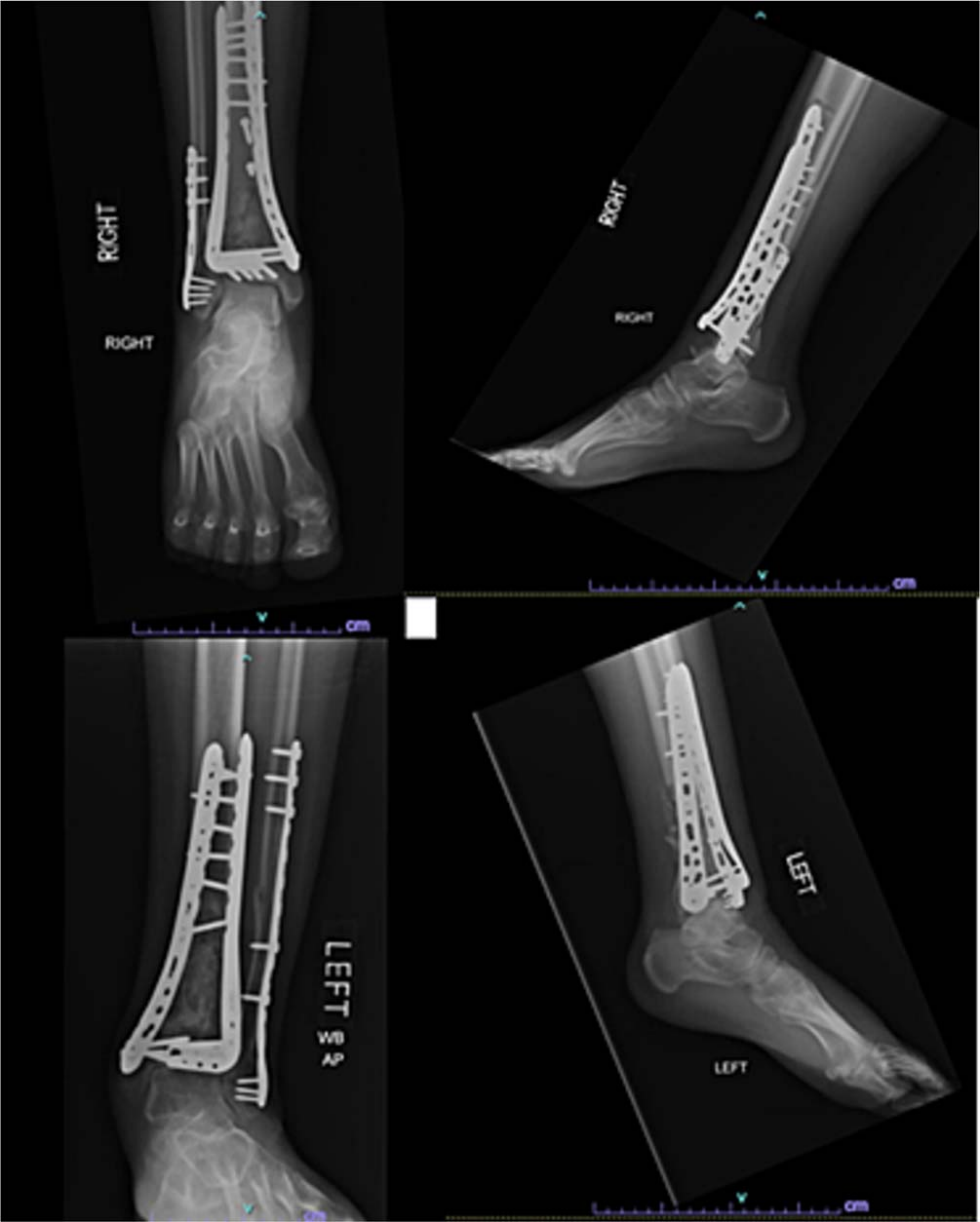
AP and lateral radiographs of patient 3 taken 3 months after posterior iliac crest bone grafting (7 months after index procedure).
